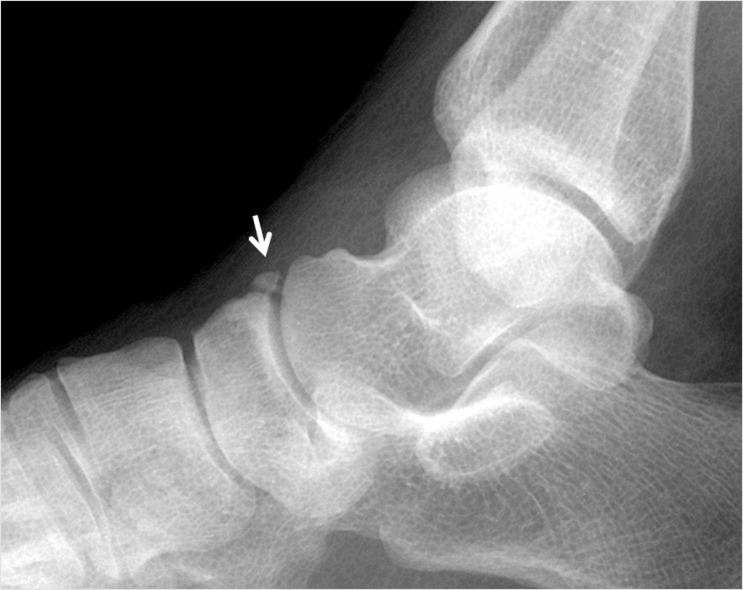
Lateral radiograph of the foot that demonstrates os supranaviculare (white arrow)
